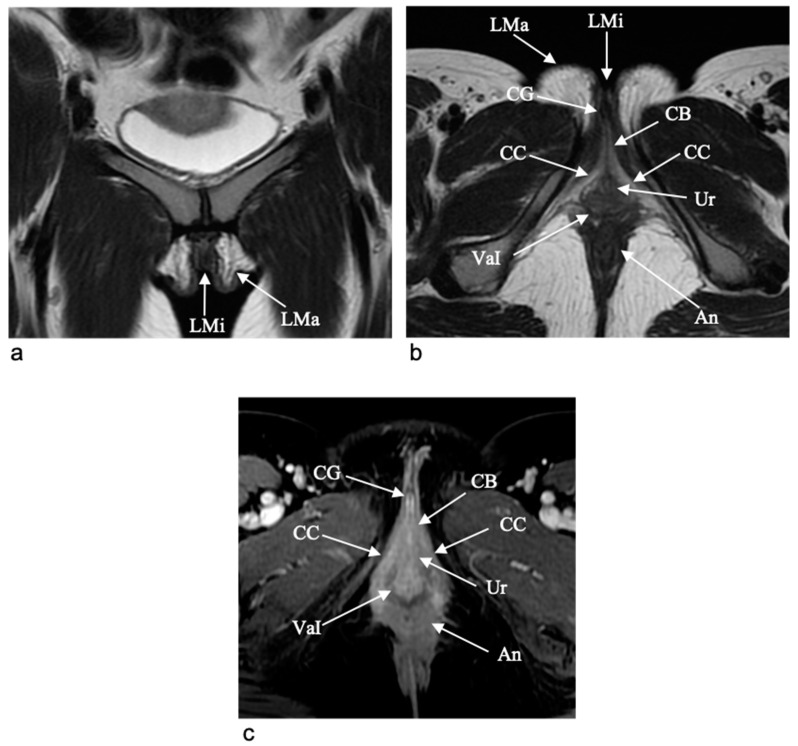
MR images of vulvar structures. On coronal (a) and axial (b) T2-W MR images the labia majora (LMa) show high signal intensity and the labia minora (LMi) show low signal intensity. On the axial T2W MR image (b) the clitoral anatomy is well evaluated with identification of the different components, such as the crura (CC), the body (CB) and the glans (CG), which show higher signal intensity than muscle and the contiguous structures. Axial contrast-enhanced T1-W MR image (c) shows a marked contrast enhancement of the clitoris; the midline septum of glans is also evident (CG). Within the labia minora there is the vestibule including the urethral meatus (Ur) and vaginal introitus (VaI). Posteriorly there is the anus (An).
